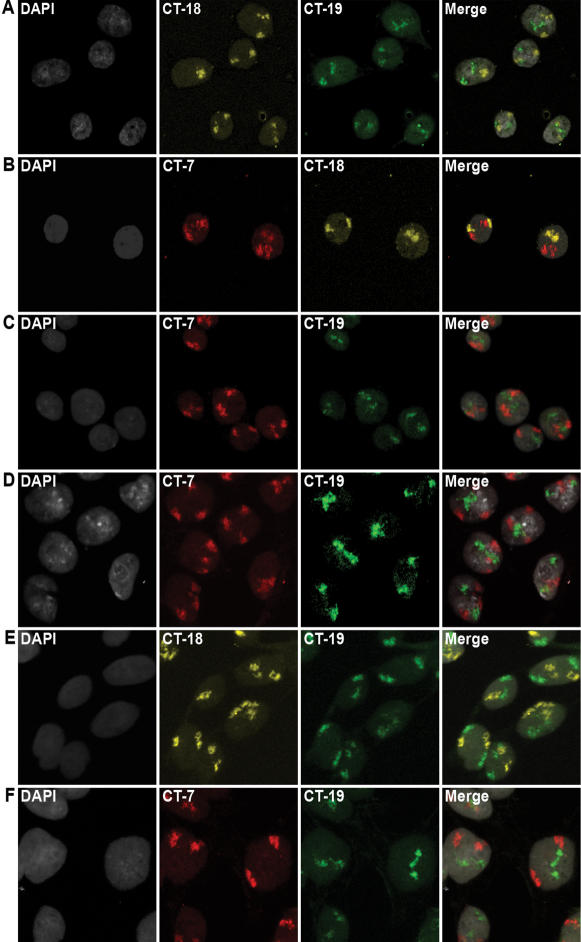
Representative maximum intensity projection of confocal image stacks from DLD-1 parental and derived nuclei. A–C: Parental DLD-1 nuclei. D: DLD-1+7 nuclei. E: DLD-1+18 nuclei. F: DLD-1+19 nuclei. DAPI: DNA counterstain; CT-7: Chromosome 7; CT-18: Chromosome 18; CT-19: Chromosome 19; Merge: merged image of DAPI and chromosome territories.
